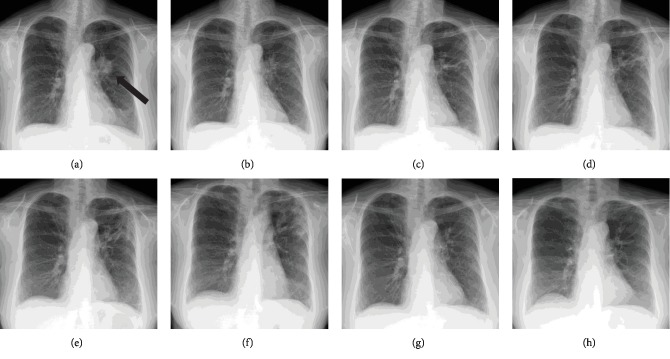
Changes in chest X-ray images. Before treatment with nivolumab (a), at the onset of CIP (b), 1 week after the onset of CIP (c), 2 weeks after the onset of CIP (d), 1 month after the onset of CIP (e), 2 months after the onset of CIP (f), 3 months after the onset of CIP (g), and 6 months after the onset of CIP (h). The relapsed lung tumor is indicated by the arrows. CIP: checkpoint inhibitor pneumonitis.
